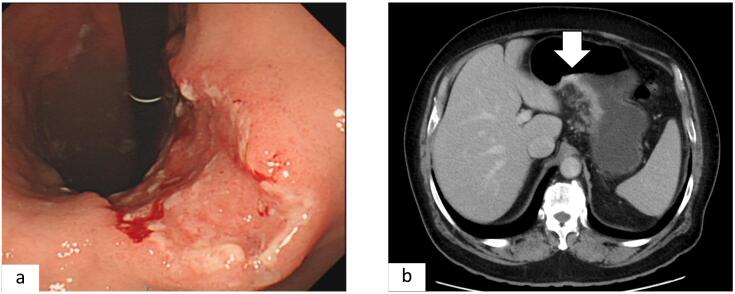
(a) EGD reexamination revealed a mass with a central ulceration at the lesser curvature extending from the gastric angle to antrum. (b) CT scans showed wall thickening with contrast effect on the anterior wall of the gastric lesser curvature and no clear distant metastases were observed.
